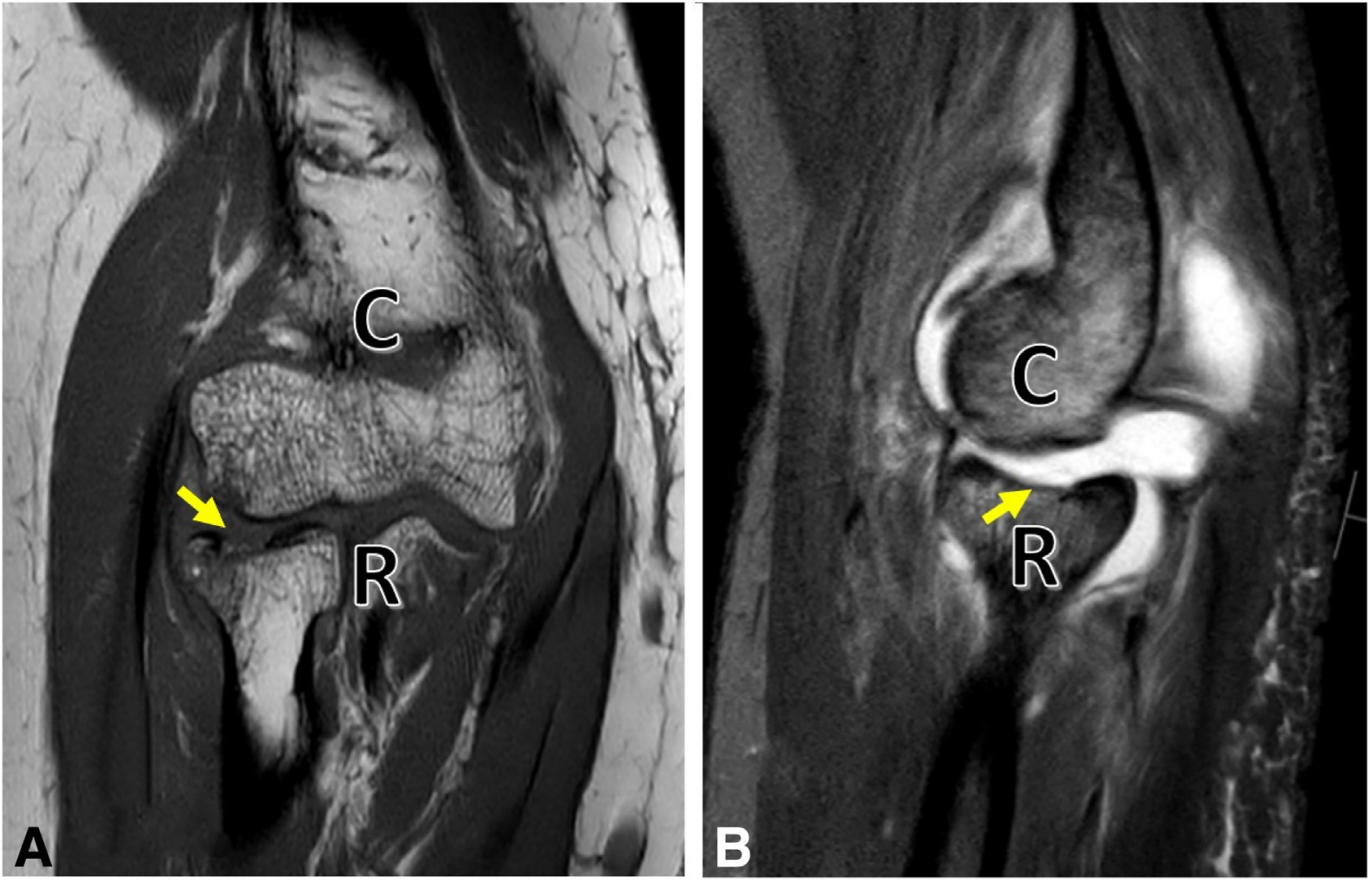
(A) Coronal and sagittal magnetic resonance imaging of the right elbow, showing the osteochondral lesion (arrow) of the radial head (R). (B) Magnetic resonance imaging of the elbow joint preoperatively with an osteochondral lesion. The present case revealed a 9-mm × 10-mm osteochondral fragment of the articular fovea of the radial head and a concomitant rupture of the lateral ulnar collateral ligament. (C, capitulum humeri.)
